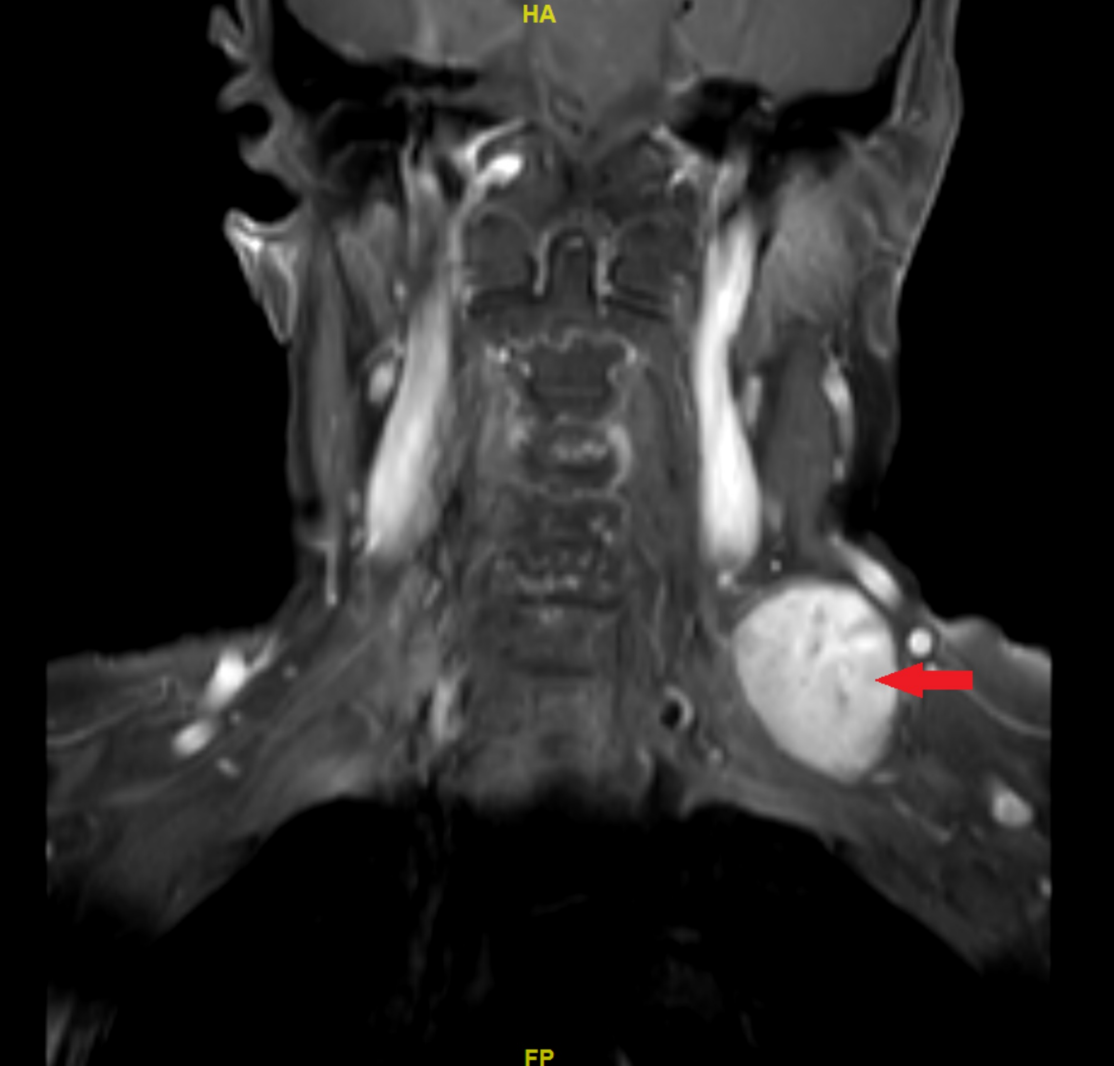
Contrasted coronal T1 MRI of the neck A left-sided well-circumscribed hyperintense neck mass (arrow) MRI, magnetic resonance imaging
